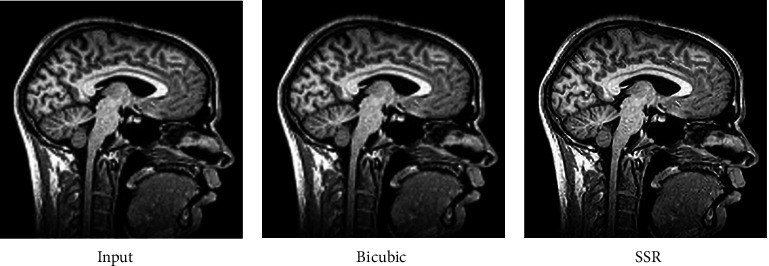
Comparison of input, bicubic, and our SSR of the CT brain.
